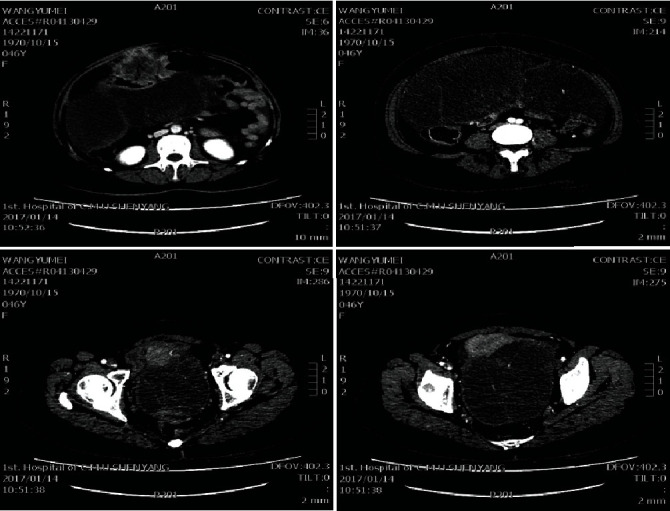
Abdominal enhanced CT shows irregular thickening of the wall of the hepatic flexure of colon with narrowing of the lumen and uneven enhancement.
